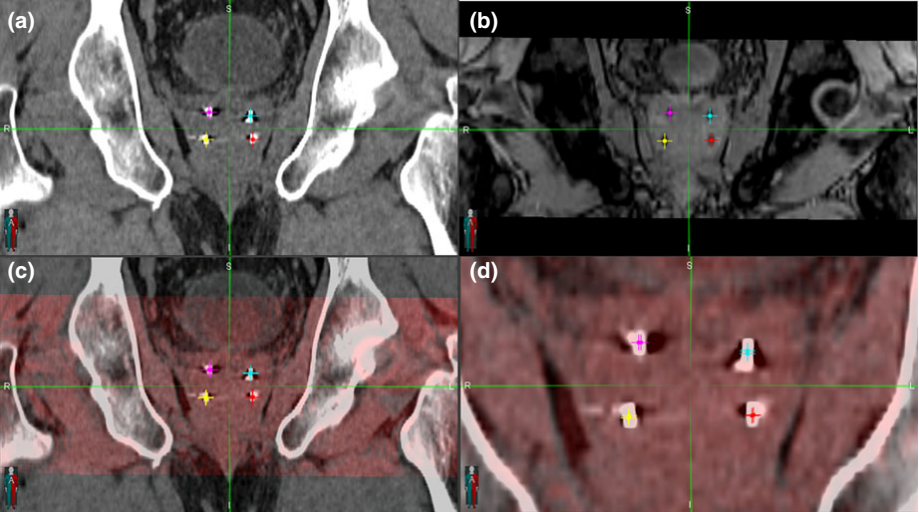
Fiducial alignment. The user‐defined location of the four fiducial markers is shown in corresponding colors on CT (a) and T2* MRI (b); (c) Alignment of the two datasets using point‐to‐point registration; (d) Enlarged view of the image in (c). demonstrating the small differences in fiducial alignment, note the two point structures can be difficult to discriminate because of their close proximity.
